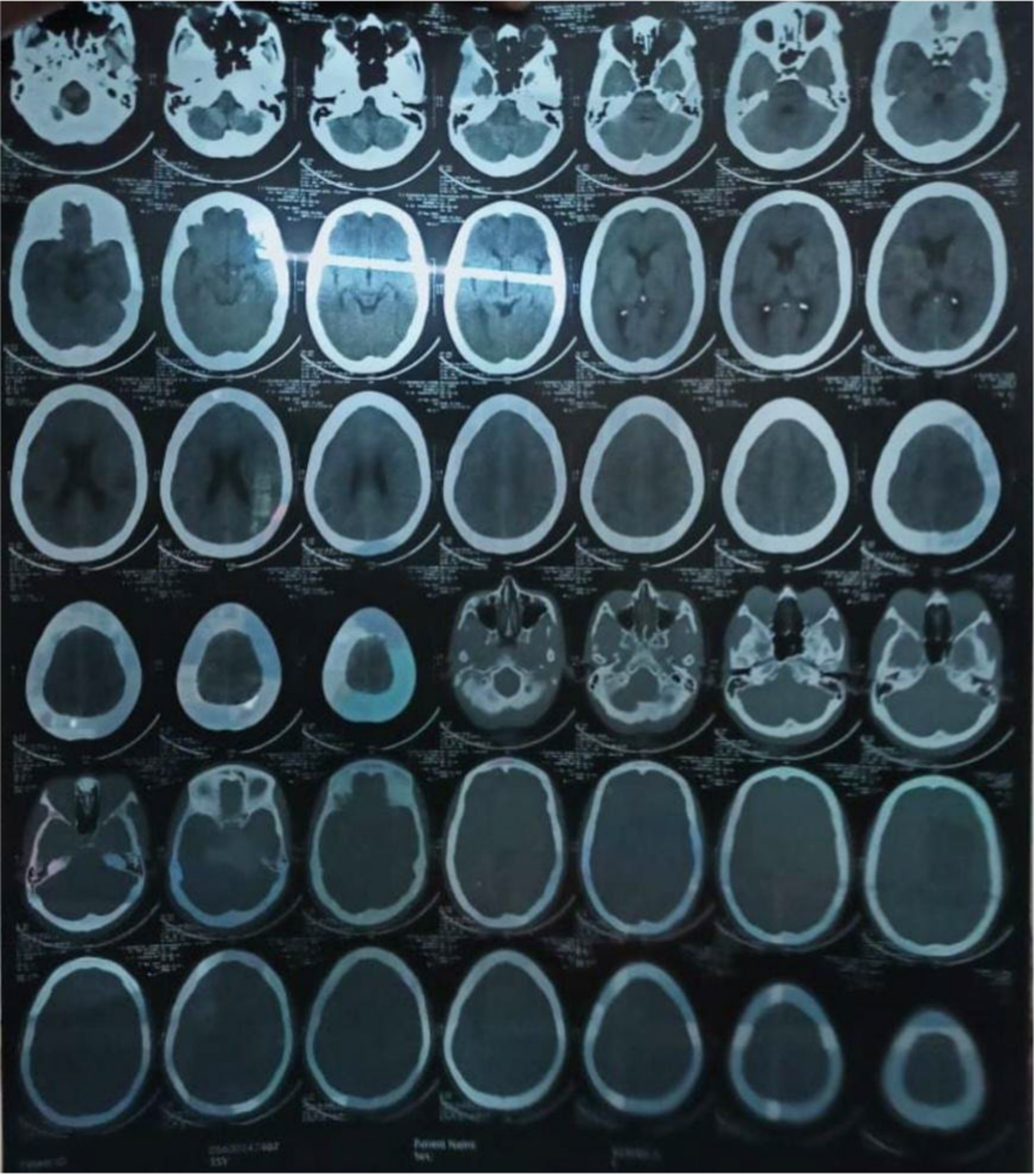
Axial sections of CT brain.
